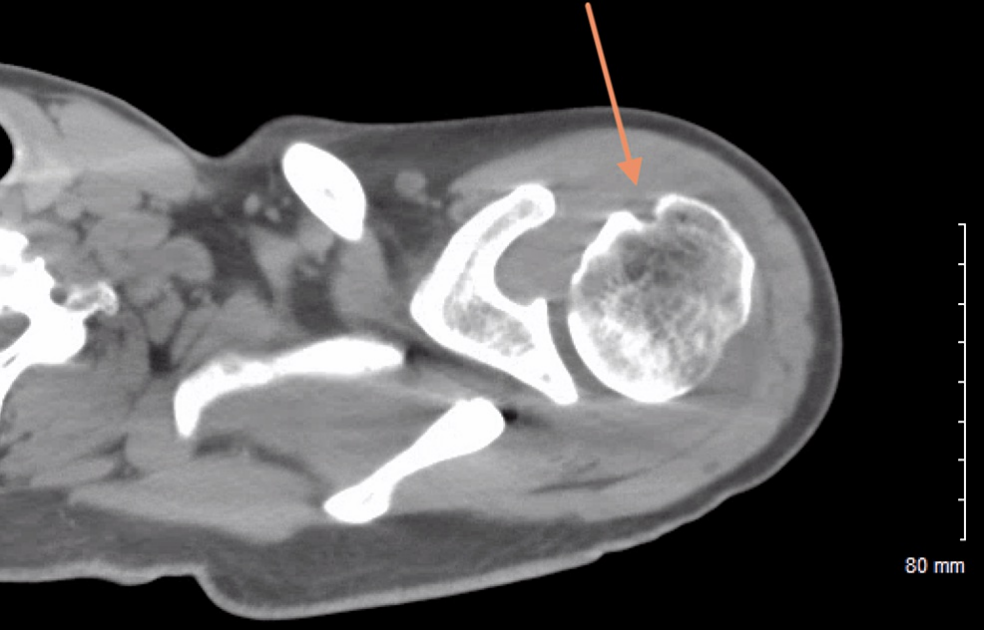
A computed tomography (CT) scan of the left shoulder Description: A CT scan with intravenous contrast of the patient’s left shoulder, demonstrating erosion at the humeral head (orange arrow) with surrounding soft tissue swelling
